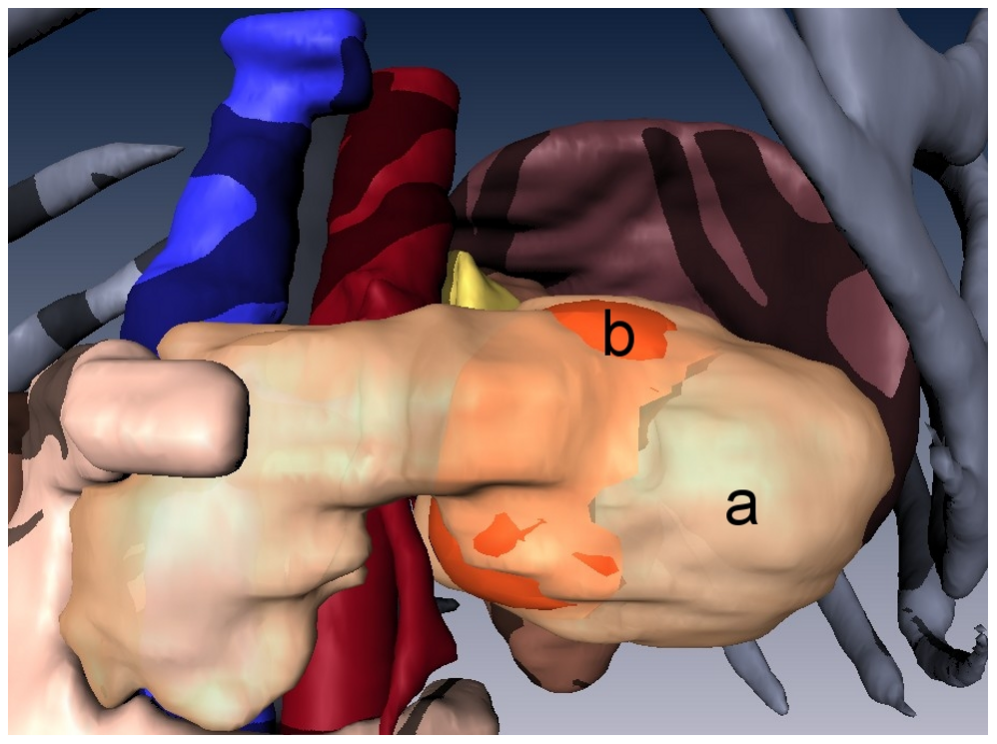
3D rendering of the ductal pancreatic mass, version A, fused with PET data. A transparent overlay of (a) the pancreas over (b) the surface rendering of the high ^18^FDG uptake region of the pancreatic tumor (orange).
